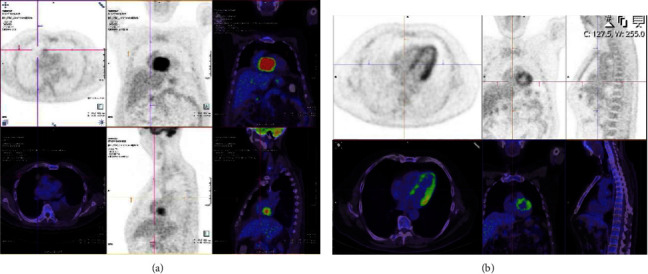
Initial PET (a) demonstrates focal intense FDG uptake corresponding to a right sided cardiac mass (SUVmax 20.6), no longer evident on progress PET (b) in keeping with complete metabolic response. There is moderate physiological uptake in the left ventricular myocardium seen in (b).
